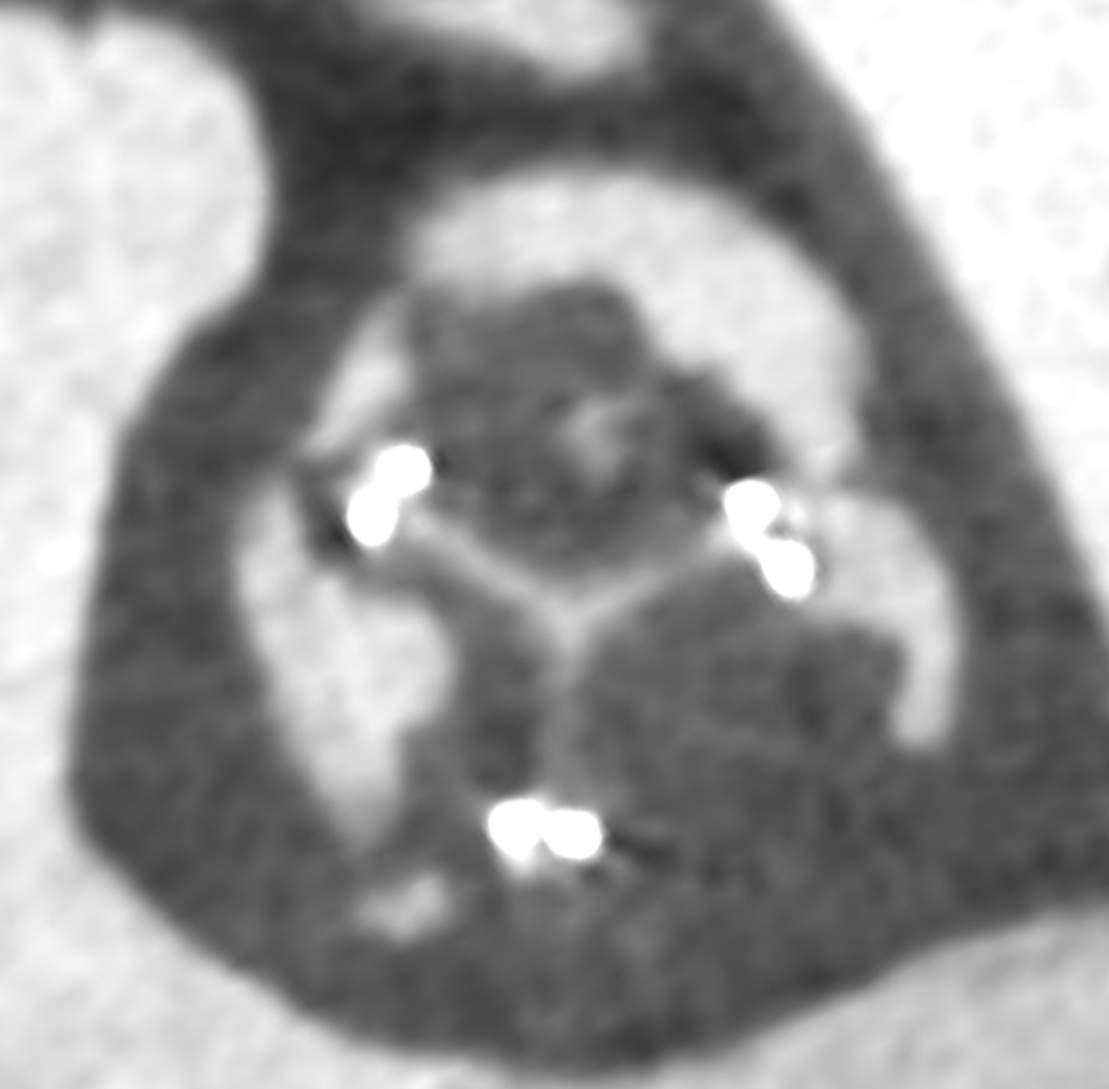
Computed tomography (CT) shows thrombi in all leaflets.
